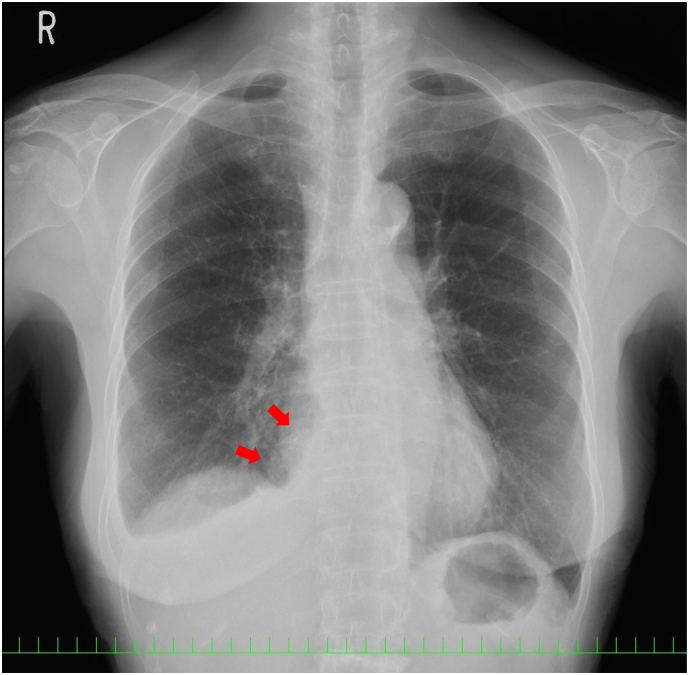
Chest roentgenogram showing a high-density mass (red arrows) in the right thoracic cavity with mild pleural effusion. (For interpretation of the references to colour in this figure legend, the reader is referred to the web version of this article.)
